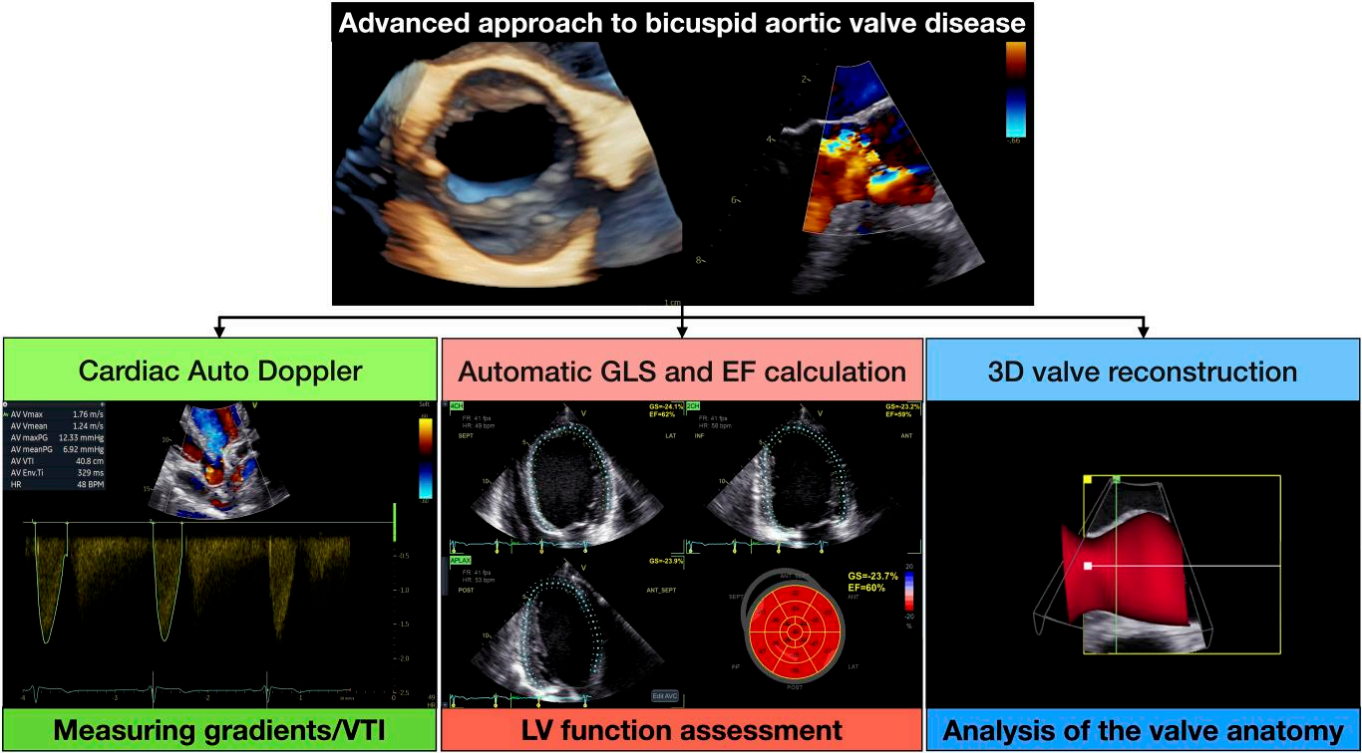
Comprehensive AI-powered approach to bicuspid aortic valve disease: Cardiac Auto Doppler to estimate gradients and velocity-time integrals (VTIs), automatic global longitudinal strain (GLS) and ejection fraction (EF) calculation to assess left ventricular (LV) function and three-dimensional (3D) valve reconstruction for a deep valve anatomical analysis.
